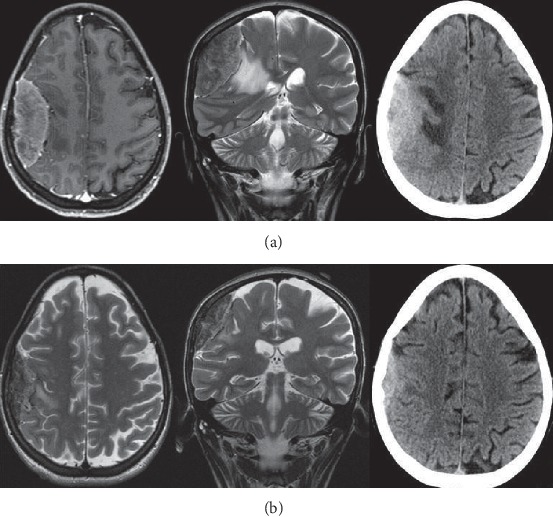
(a) MRI study with enhancement documented right fronto-parietal dural-based extra-axial mass causing moderate mass effect. The lesion was isointense on T1-weighted and hyperintense on T2-weighted images, with evidence of dural tail. (b) A mass reduction at CT-scan and MRI studies after 1 week of corticosteroid therapy.
